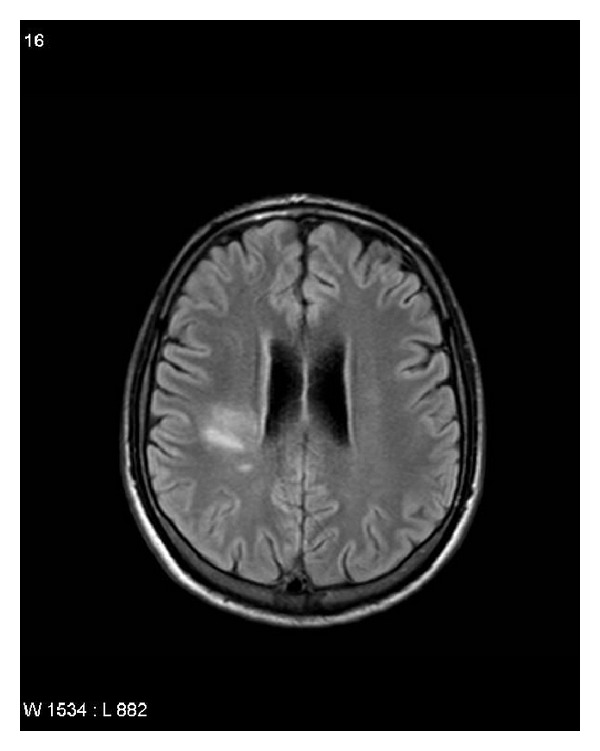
An emergency brain angio-computed tomography (A-CT) evidenced thrombosis of the M2 tract of the right middle cerebral artery.
